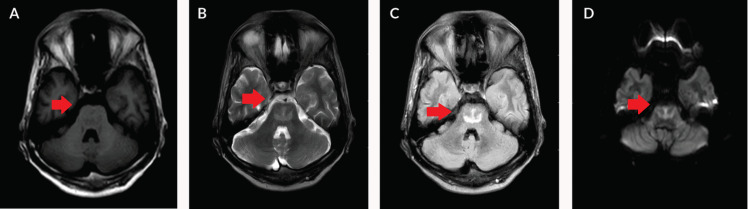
Evidence of altered signal intensity lesion noted in the pons appearing hypointense on T1WI (A), hyperintense on T2WI/FLAIR (B, C), showing diffusion restriction on DWI (D) in the axial section FLAIR: fluid-attenuated inversion recovery; DWI: diffusion-weighted imaging
